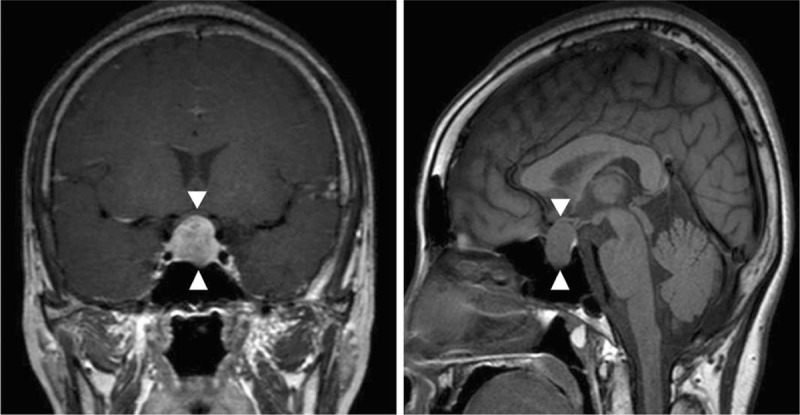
Pituitary macroadenoma with suprasellar extension detected on contrast-enhanced magnetic resonance imaging.
